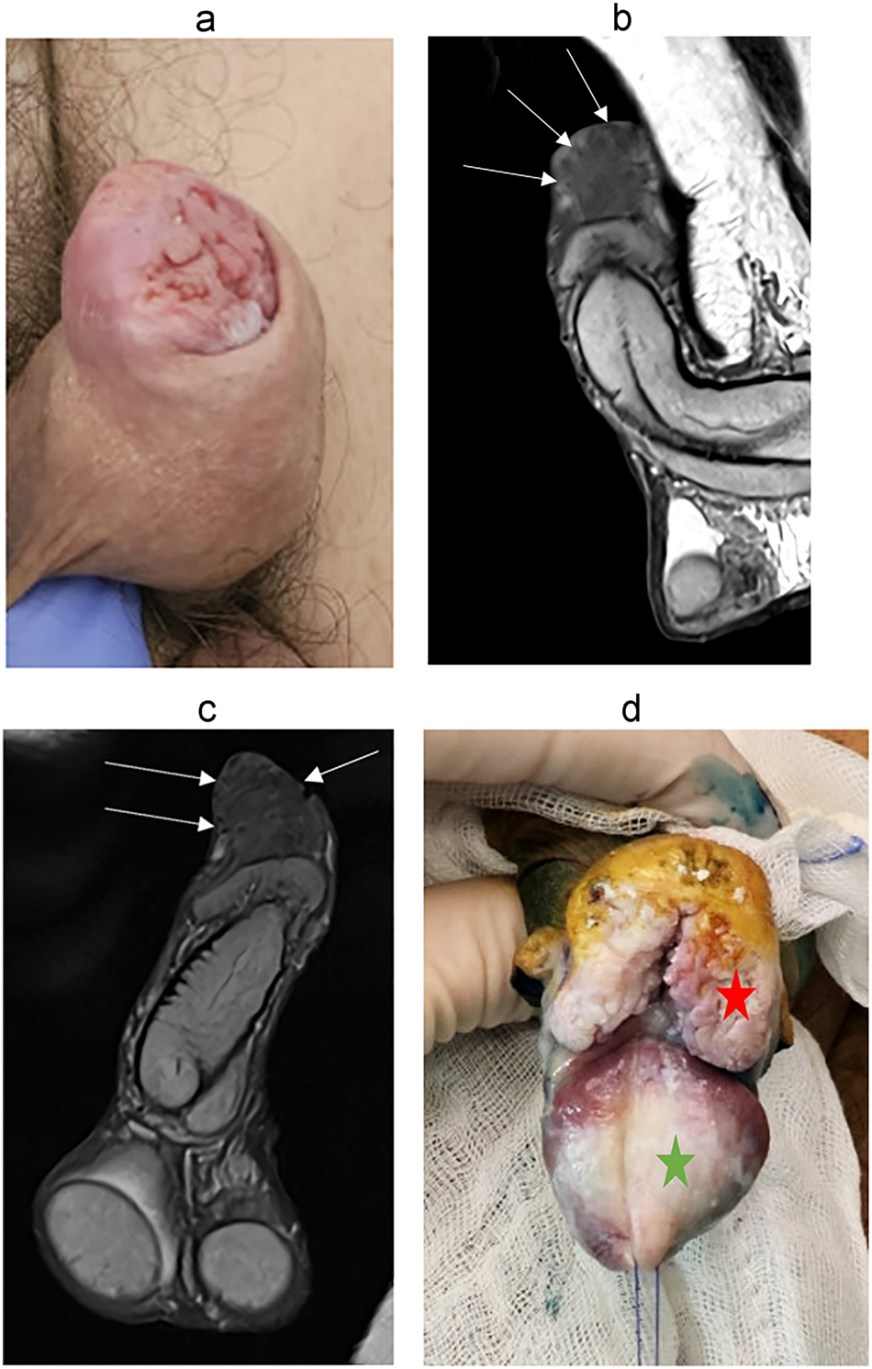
Seventy-two-year-old patient, referred for a malignant-looking tumor on the dorsal part of the foreskin, which comes into contact with the glans and appeared fixed (a). b Sagittal, (c) coronal T2-W MR images show a large budding tumor above the glans, without extension to the corpus spongiosum (arrows). Patient underwent posthectomy showing a well differentiated keratinizing squamous cell carcinoma grade 1 of the foreskin, non HPV associated, limited to the connective planes of the foreskin. Stage pT1N0. Intraoperative image (d) showing the tumor excision (red star). The glans appears healthy (green star)
